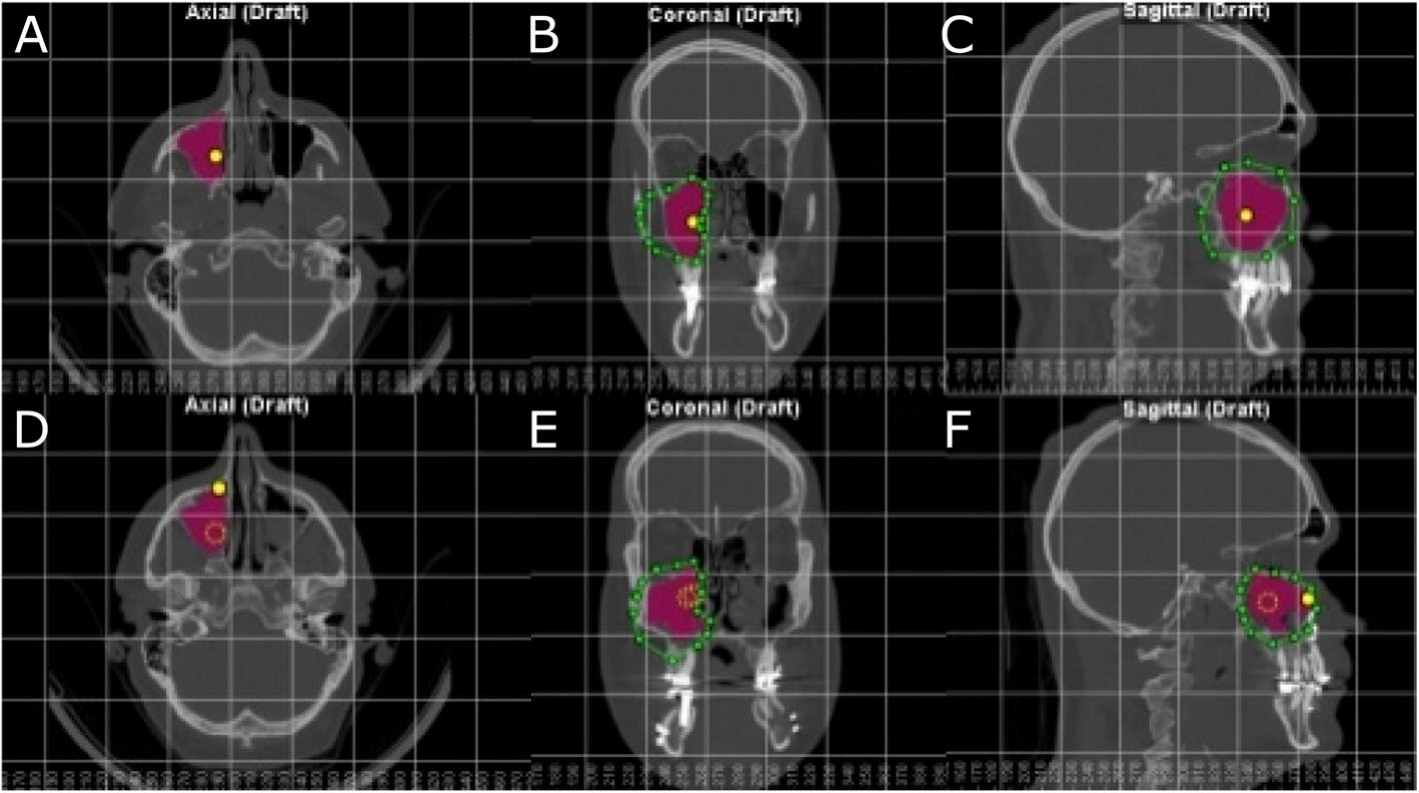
A Axial, (B) coronal and (C) sagittal plane of the preoperative CBCT scans and (D) axial, (E) coronal and (F) sagittal plane of the postoperative CBCT scans. Green line describes the selected area, yellow points define the air density and purple area describes the volume of the left maxillary sinus
